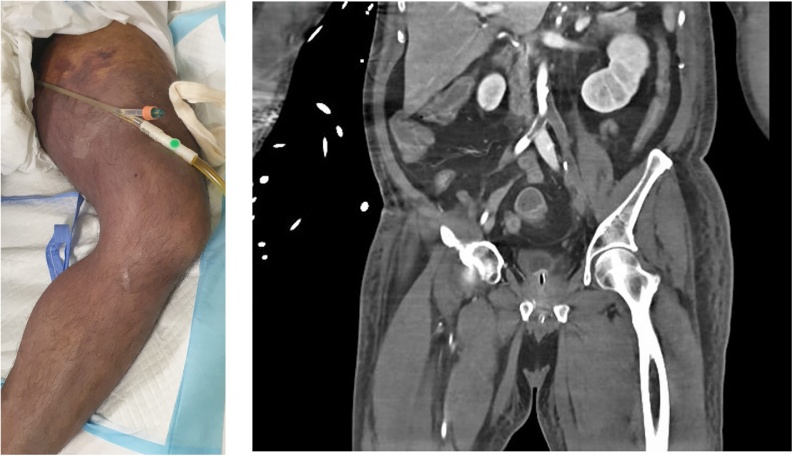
(a) Left lower limb with visible mottled and dusky appearance. (b) CT angiography, red arrow displaying significant occlusion aortic bifurcation and left common iliac artery.
